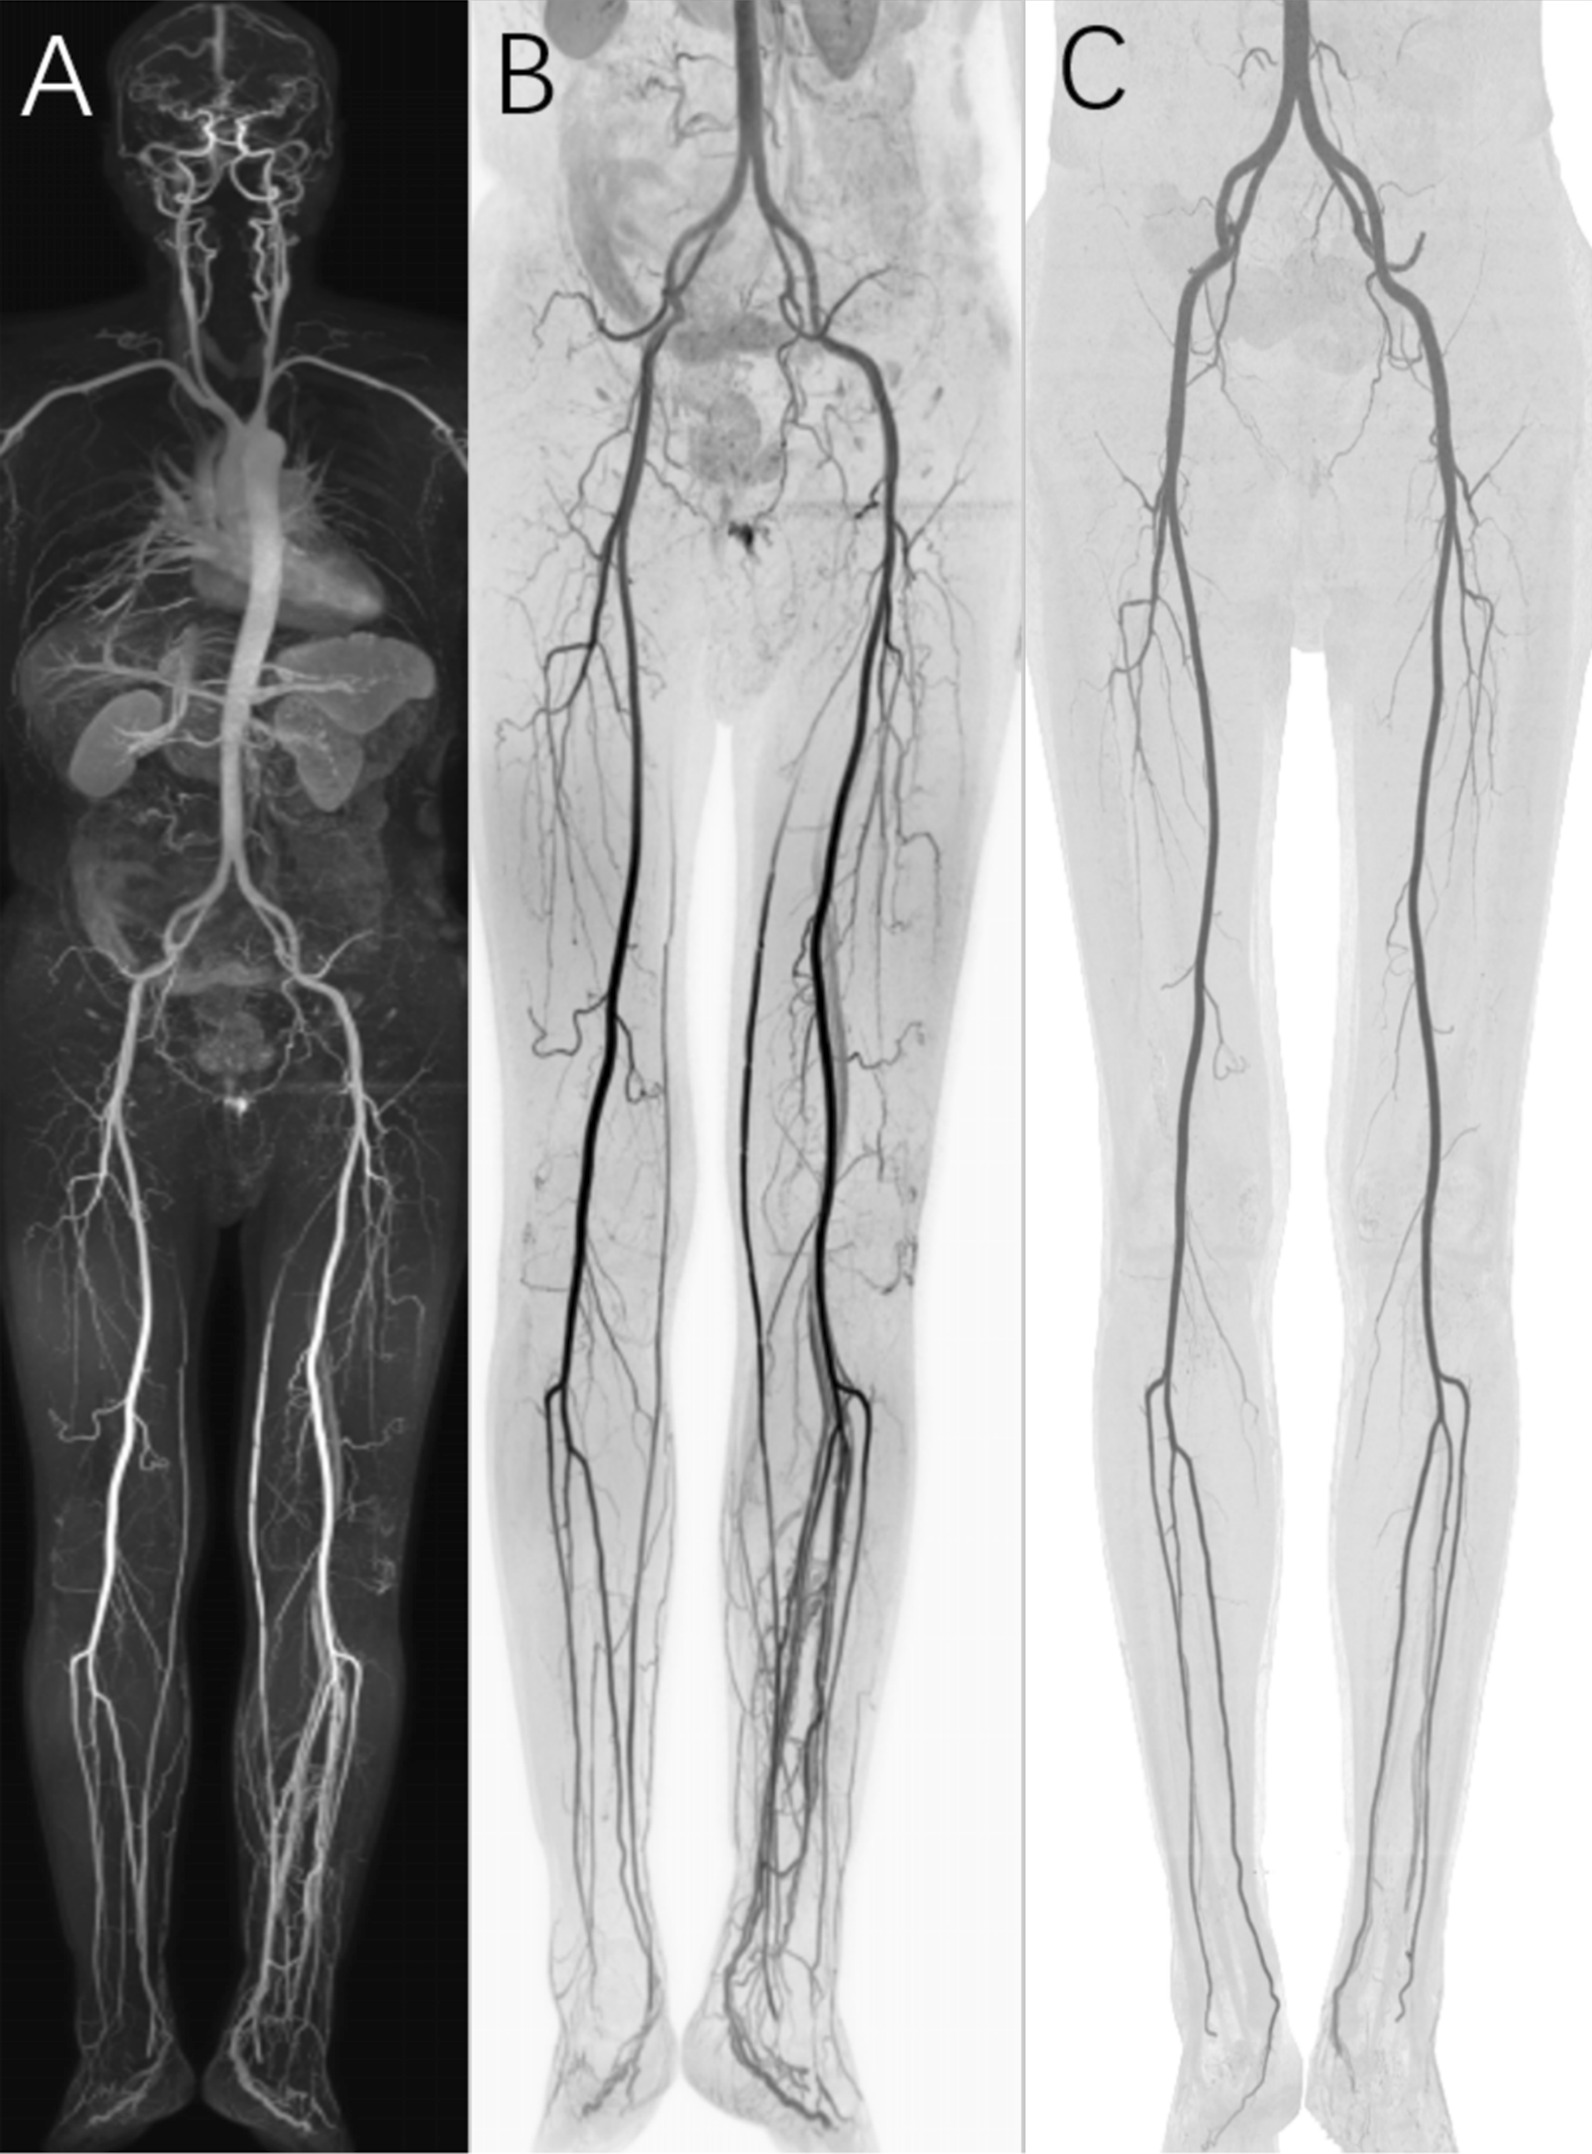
A male patient, 69 years old, presented with numbness in both lower limbs for a month. Whole-body subtractionless CS-WBMRA MIP (A) and inversed MIP of the lower extremity (B) showed normal arterial vascular tree of the whole body with early enhanced left calf vein. Inversed lower extremity CTA (C) confirmed the reliability of CS-WBMRA data
